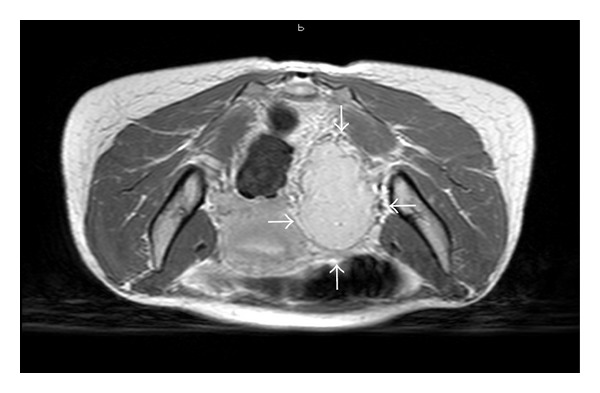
Pelvic MRI suggestive of a parauterine left site extra-adrenal sympathetic paraganglioma in the images obtained after i.v. injection of gadolinium: axial view.
